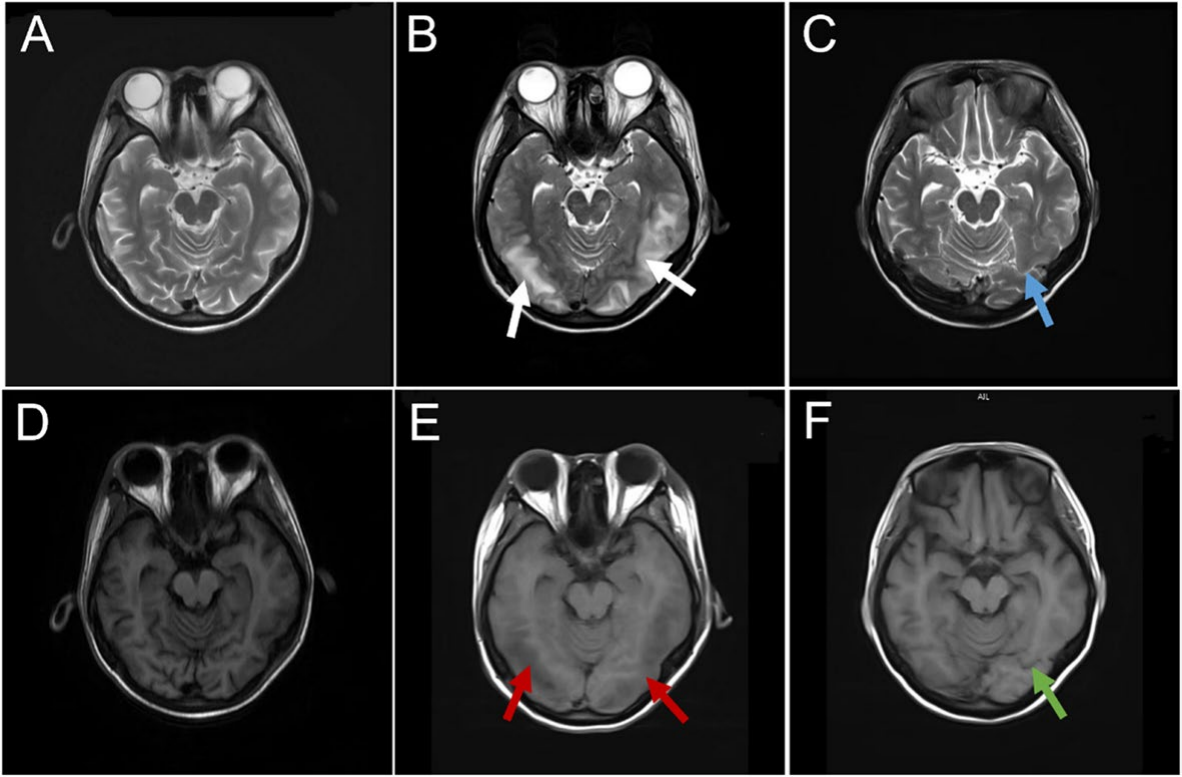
Magnetic resonance imaging brain findings. A D Before the onset of encephalopathy. B E Encephalopathy. MRI brain with T2 and T1 weighted images showed hyperintensity (white arrows) and hypointensity (red arrows) in bilateral temporoparietal subcortical areas, respectively. C F After treatment and reexamination. Hyperintensity (blue arrow) and hypointensity (green arrow) in temporoparietal subcortical areas disappeared
